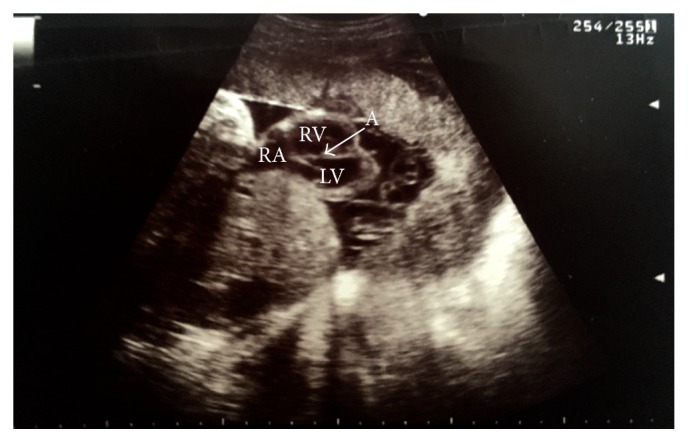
Ultrasound of fetus heart at 42nd week of gestation with ventricular septal defect. RA: right atrium, RV: right ventricle, and LV: left ventricle. A: ventricular septal defect.
